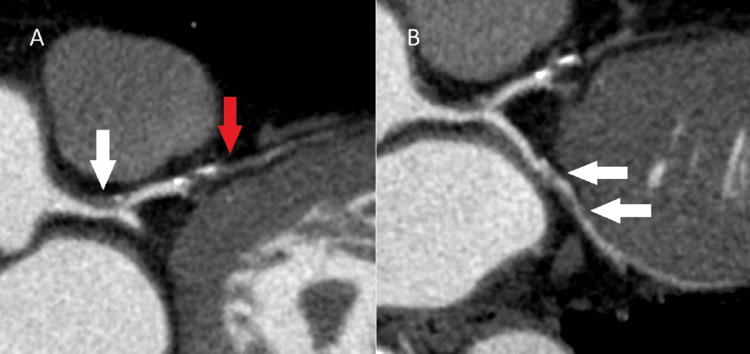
Curved multi-planar reformation of preoperative contrast computed tomography (A) Significant stenosis (white arrow) of the left main trunk and complete occlusion (red arrow) of the left anterior descending artery were suspected. (B) Significant stenosis (white arrows) of the circumflex artery was suspected.
